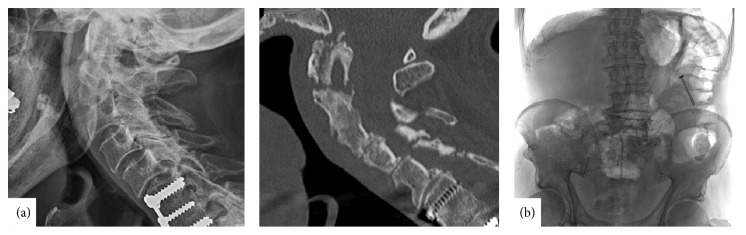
(a) X-ray (left) and computed tomography (CT) (right) show missing odontoid screw and not consolidated fracture gap, sagittal view. (b) Abdomen X-rays displaying an odontoid screw in the left upper abdomen, presumably descendent colon, with no signs of free peritoneal air, a.p. view.
